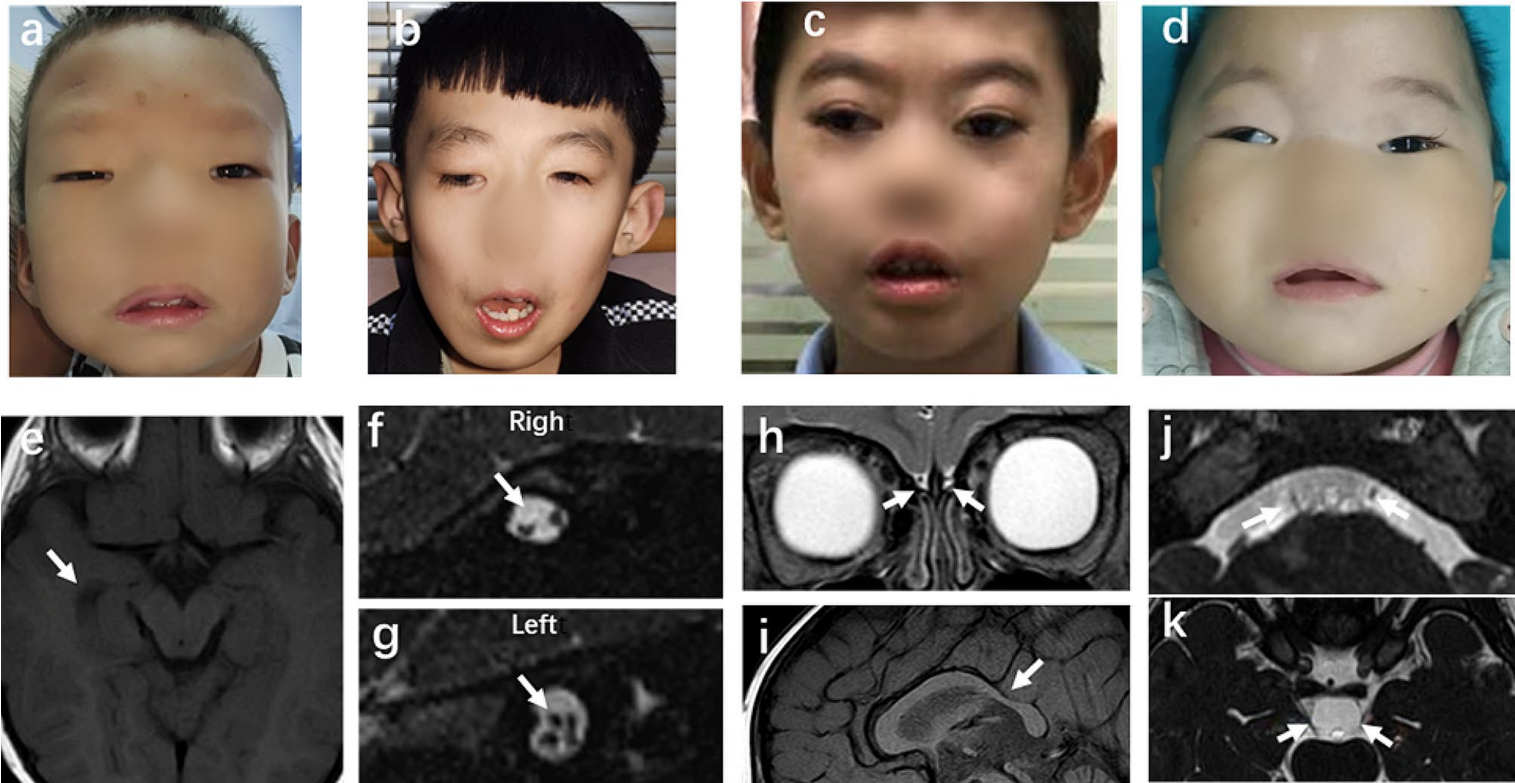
Ocular and MRI findings of four patients with the *TUBB3* Glu410Lys variant. a–d. Facial photographs of patients S16 (a), S17 (b), S26 (c), and S27 (d), showing bilateral blepharoptosis and facial weakness in patients S16, S17, and S27 (patient S26 had undergone correction of blepharoptosis). e. Enlargement of the lateral ventricle on its temporal horn in patient S16. f, g. Absence of the right CN7 in patient S16. h.i. Hypoplasia of the corpus callosum and the bilateral olfactory bulbs and sulci in patient S17. j. Hypoplasia of the bilateral CN6 in patient S26. k. Hypoplasia of the bilateral CN3 in patient S27
